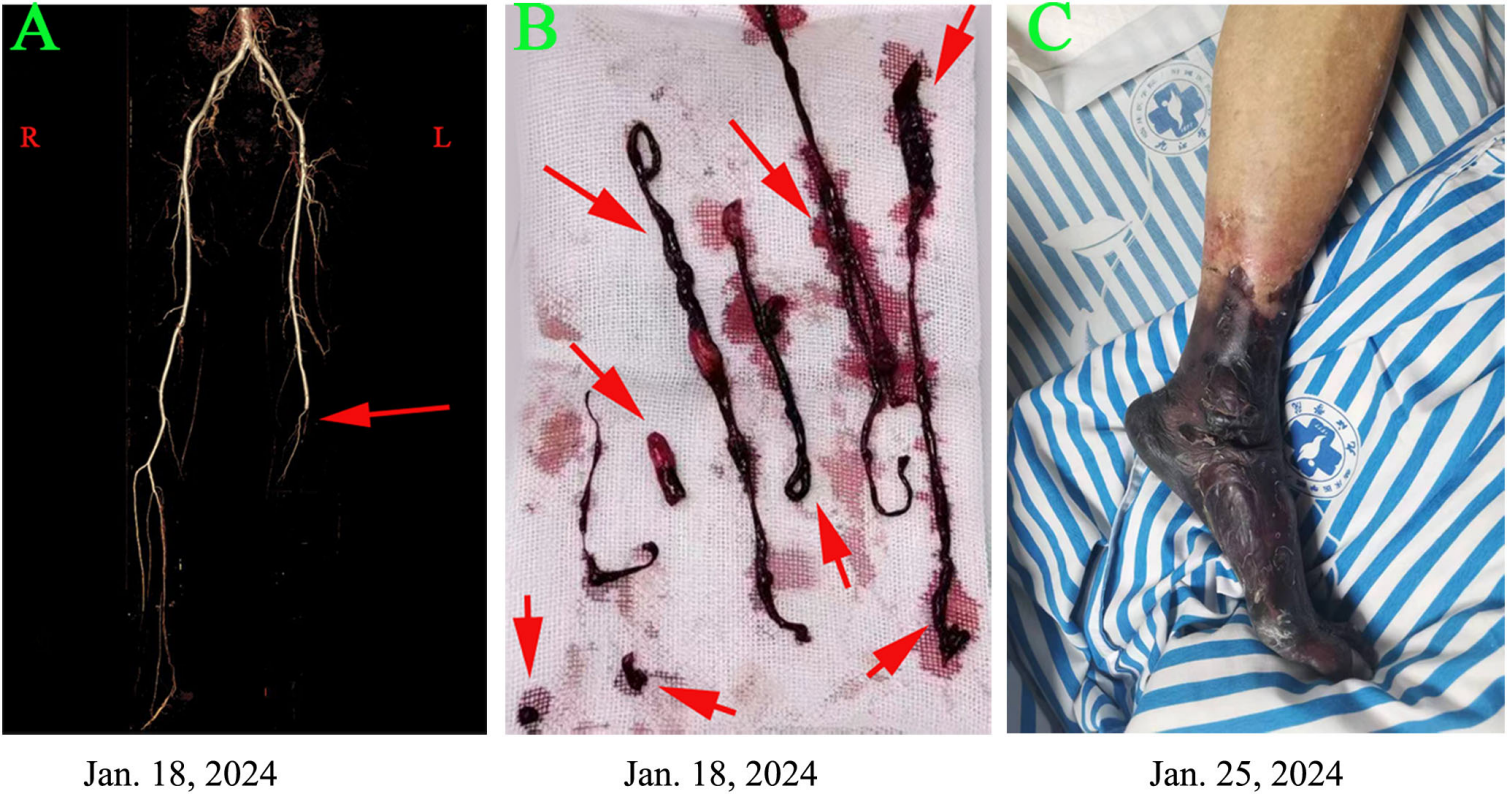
Clinical and imaging findings are presented in the patient with a popliteal artery tumor thrombus as follows: (A) Computed tomography angiography on January 18, 2024, revealed complete occlusion distal to the left popliteal artery. (B) A salvage percutaneous thrombectomy was performed on the same day, successfully retrieving multiple thrombi. (C) Despite these interventions, severe limb ischemia progressed, leading to gangrene in the left leg by January 25, 2024.
